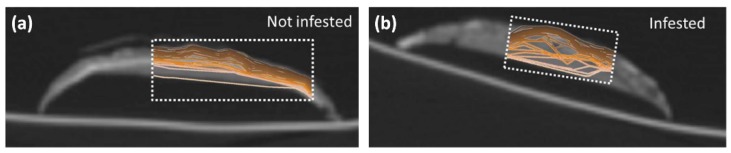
THz scans overlaid on CT images, revealing beetle damage. a) Healthy wood; b) infested wood. Reprinted with permission from [205] © The Optical Society.
